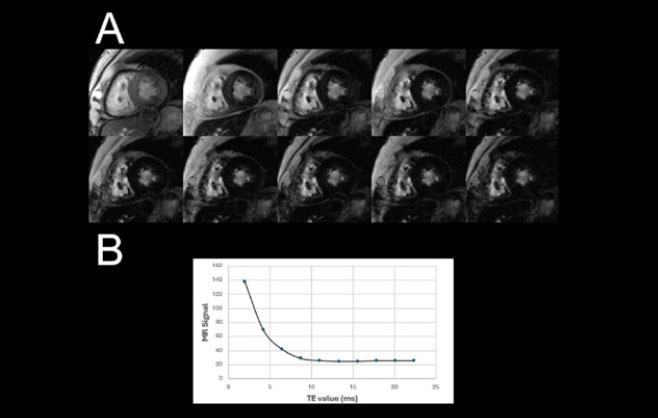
In A, Cardiac MRI using a multi-echo GRE (T2*) sequence
